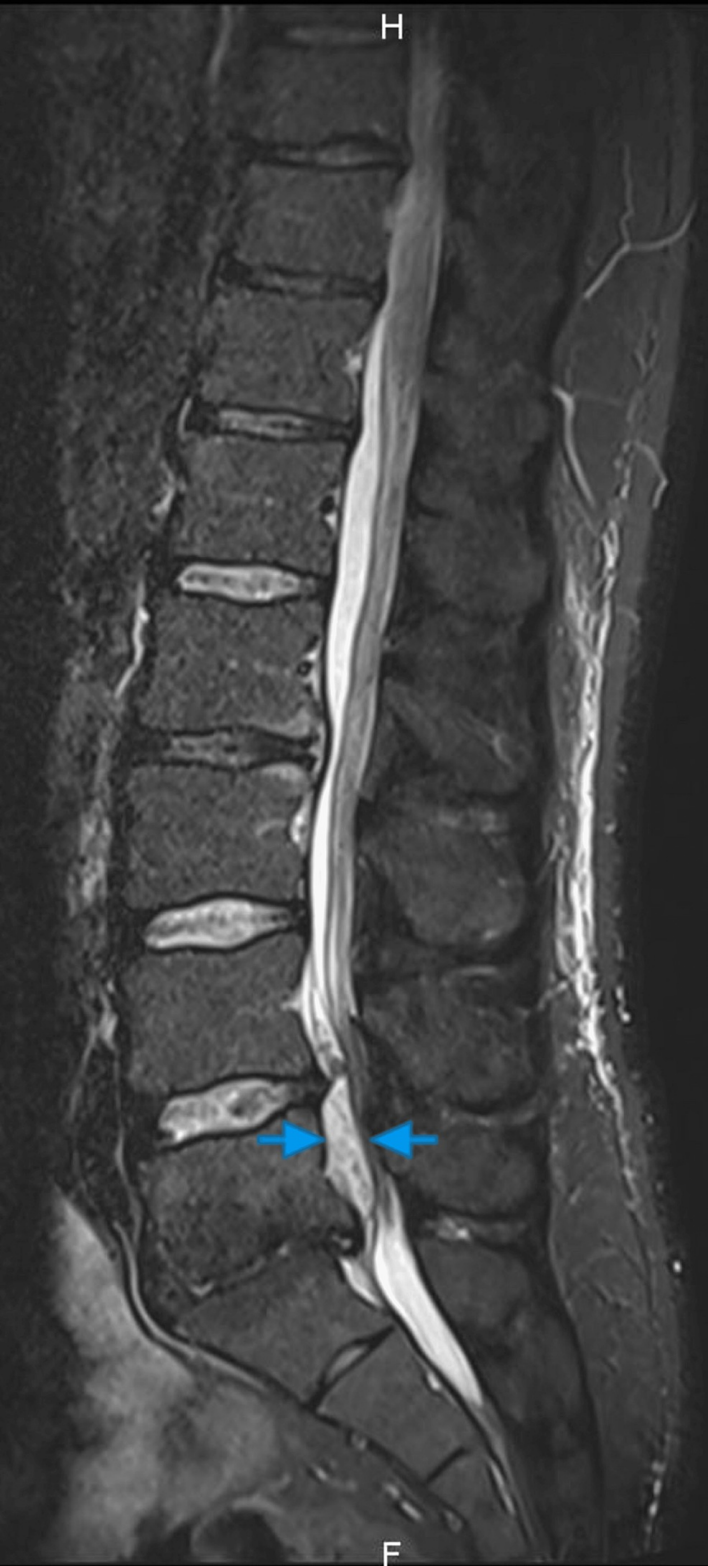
Sagittal T2-weighted fat-saturation image. Sagittal T2-weighted, fat-saturation image showing spinal cord compression by a mixed intensity structure at L4-L5 level. The structure is delineated by two blue arrows on each side.
